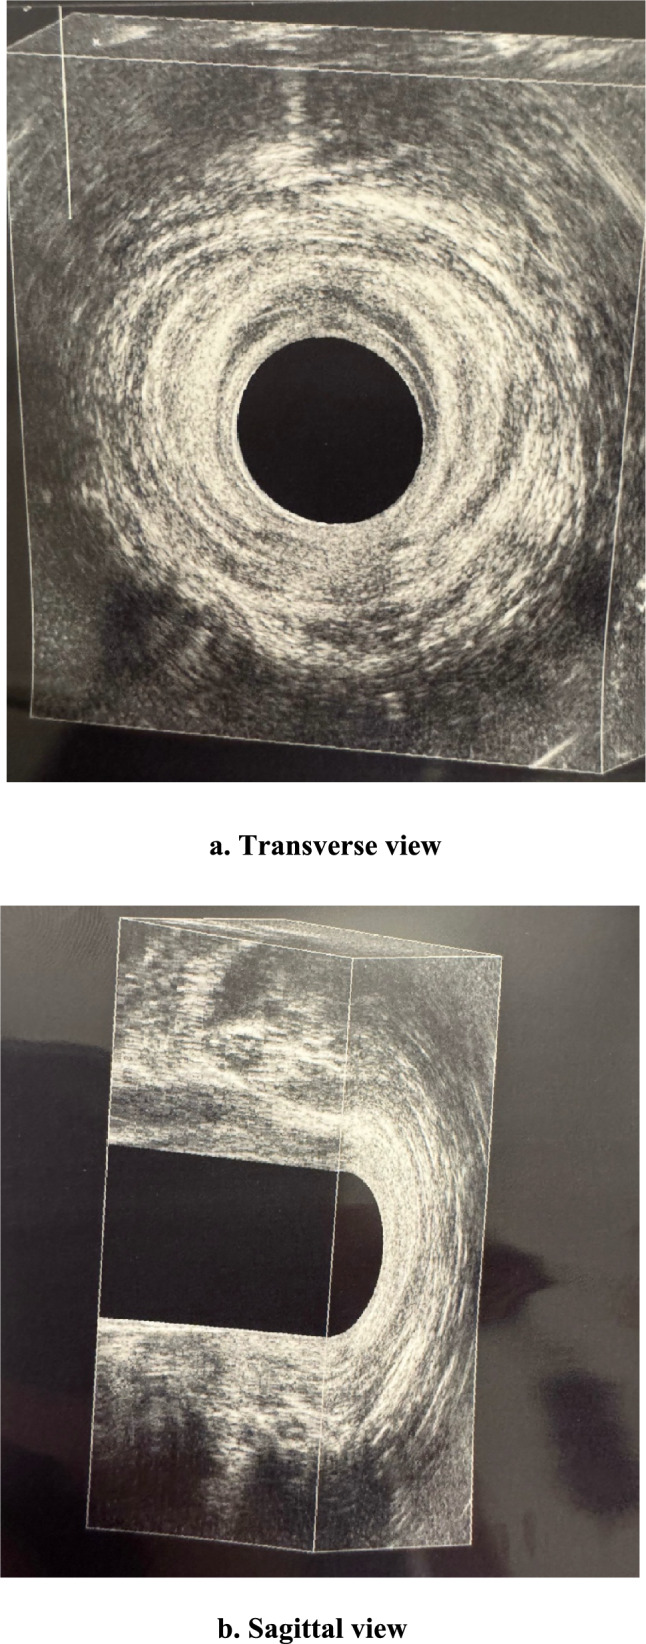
A 6-month endoanal ultrasound showing the presence of homogeneous tissue of intermediate echogenicity, without active fistulas or inflammatory collections, located posteriorly (6 o’clock). a Transverse view. b Sagittal view
